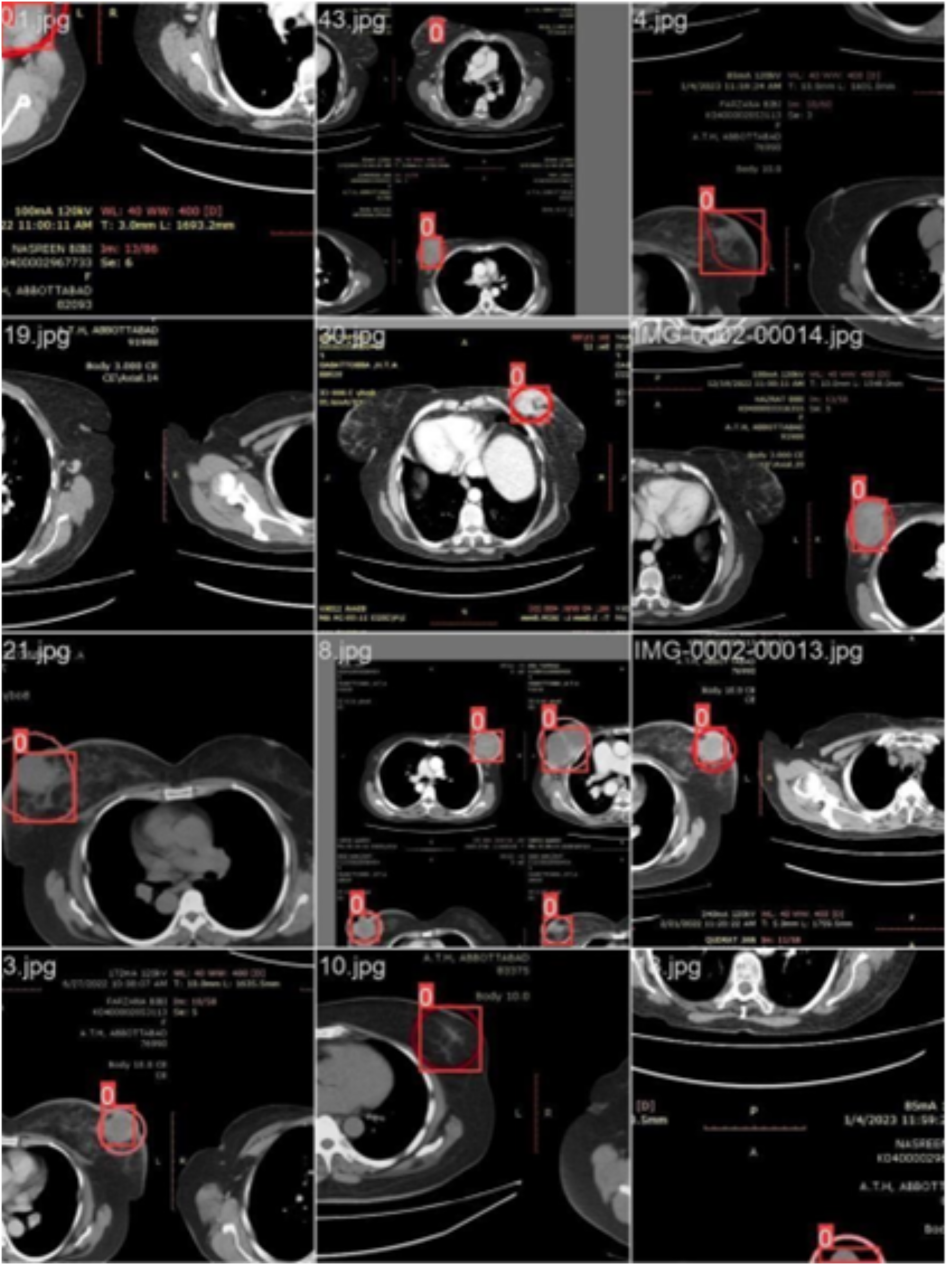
CT scans of breast cancer from 16 patients with the disease, including training samples from batch 2.
